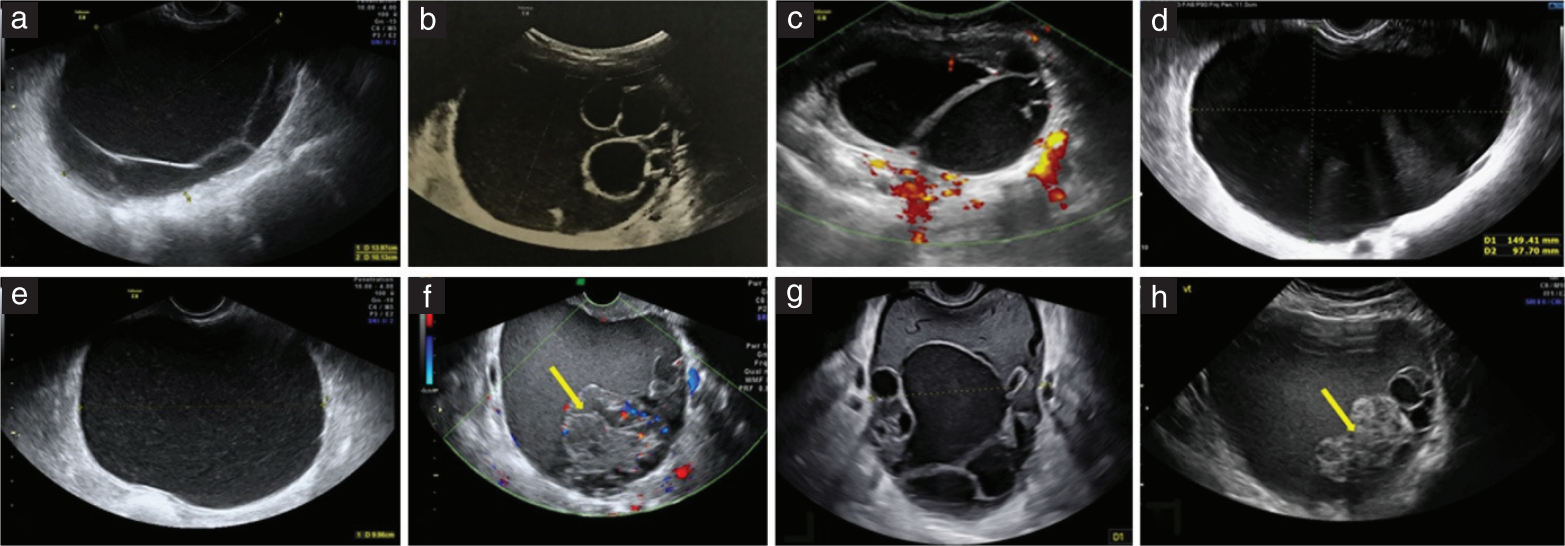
Grayscale (a,b,d,e,g,h), power (c) and color Doppler (f) ultrasound images showing typical features of mucinous cystadenomas. Most mucinous cystadenomas were multilocular cysts with < 10 locules (a–c), unilocular cysts (d,e) or multilocular cysts with > 10 locules (f–h). A honeycomb nodule can be seen in (f) and (h) (arrows).
